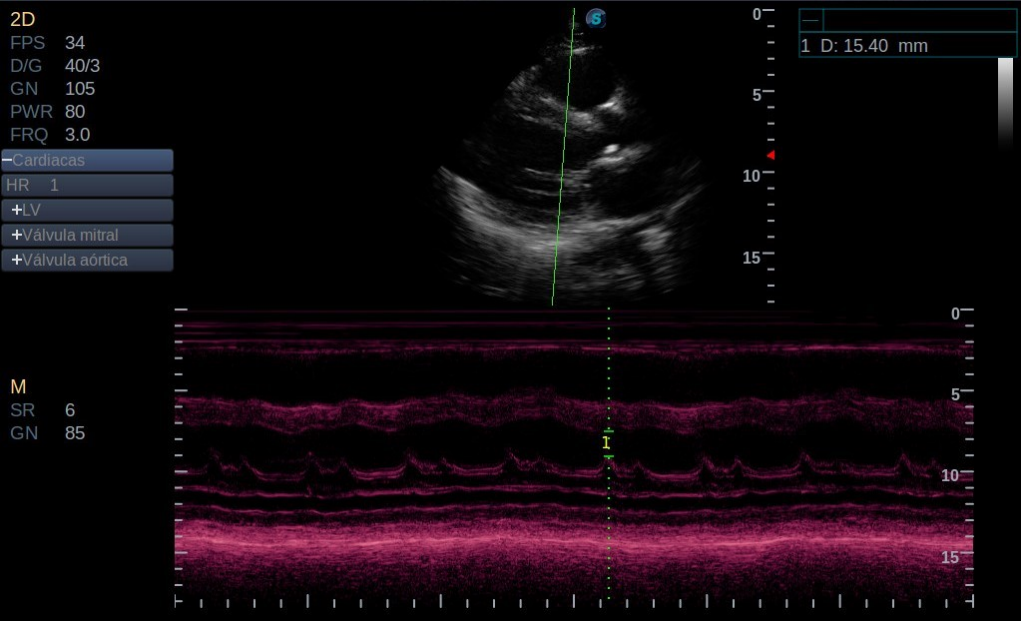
E-Point Septal Separation Measure. The E-Point Septal Separation measure was taken in early diastole, using Parasternal Long Axis View (PLAX) M-Mode, between the tip of the anterior mitral valve leaflet and the interventricular septum.
